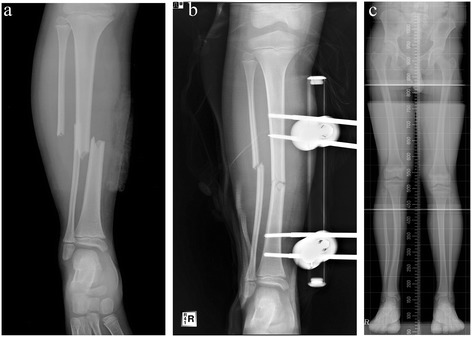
Serials radiographs of a nine-year-old boy who sustained a right distal tibiofibular fracture. (a) Anteroposterior radiograph at trauma. (b) Immediate postoperative radiograph. Treatment involved external fixation of the tibia. The postoperative angulation was 10° valgus. (c) A standing orthoroetgenogram after 5 years shows persistent valgus angulation.
